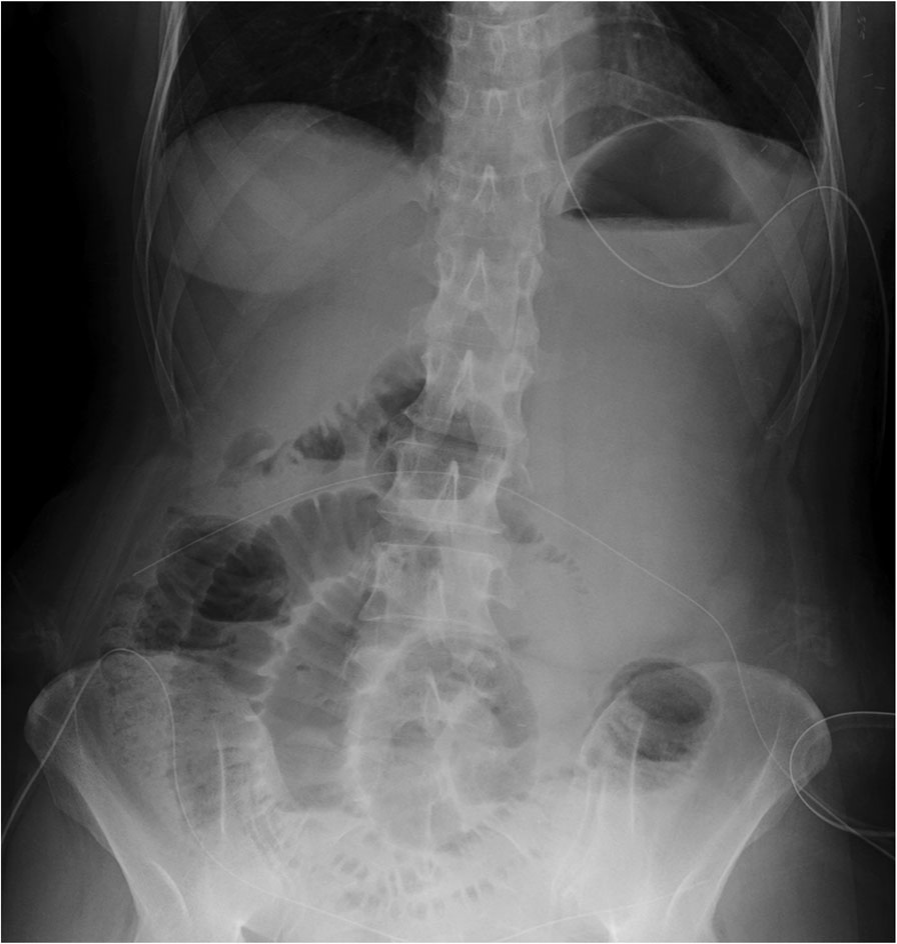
Abdominal X-ray examination showing niveau sign
